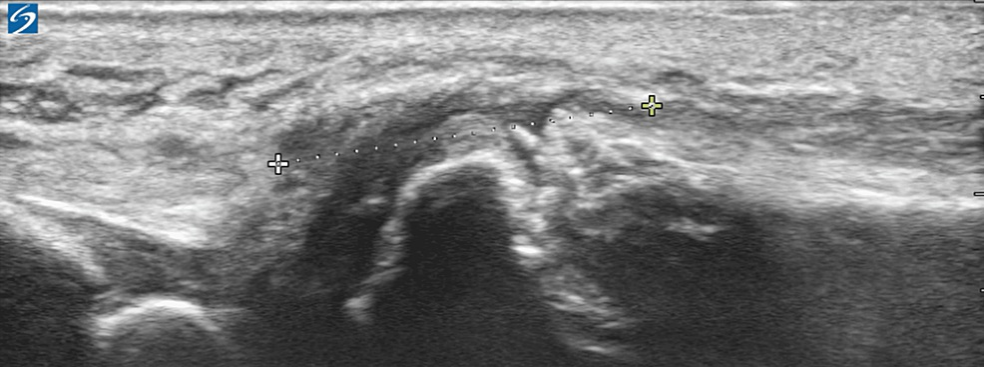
Ultrasound image demonstrating a complete EDM rupture with a 2cm gap and a partial EDC tear. EDM: Extensor digiti minimi tendon' EDC: Extensor digitorum communis.
